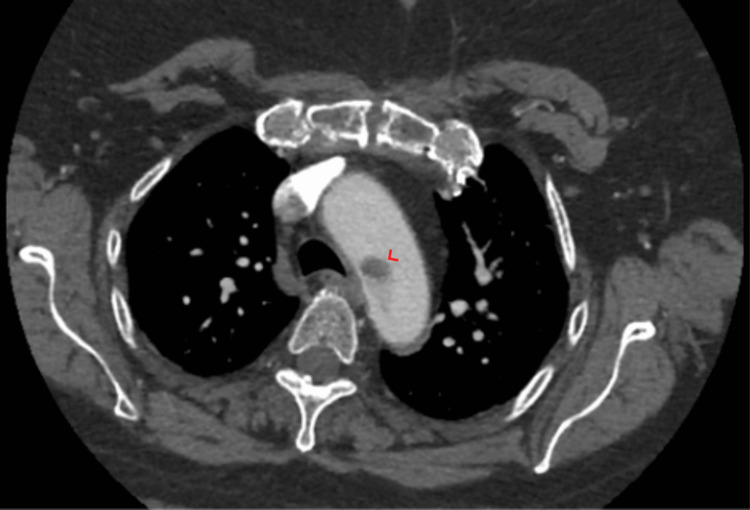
CTA/Aortogram showing a non-occlusive distal aortic arch filling defect (arrowhead). CTA: computed tomography angiogram
